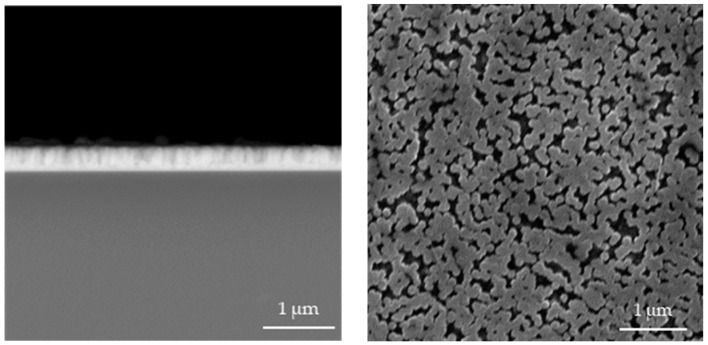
SEM images of nanorods obtained with 5 min of ultrasound and dried for 1.5 h (left: cross-section image; right: surface image).
